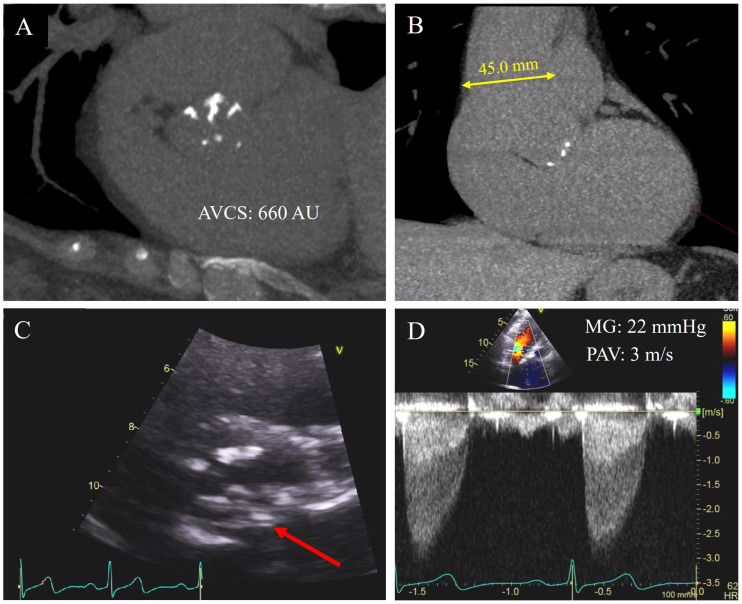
A 62-year-old asymptomatic female sent for coronary artery calcium (CAC) score for risk stratification for statin therapy. The CAC score was 0, but significant aortic valve calcification was identified (A). AVCS was quantified as 660 AU. She was also incidentally noted to have mid ascending aorta dilation with diameter of 45 mm (B). Subsequent echocardiogram for further evaluation confirmed a bicuspid aortic valve with raphe between the left and non-coronary cusp, demonstrated by arrow (C), and overall moderate aortic stenosis with mean gradient (MG) of 22 mmHg and peak aortic velocity (PAV) of 3 m/s (D).
